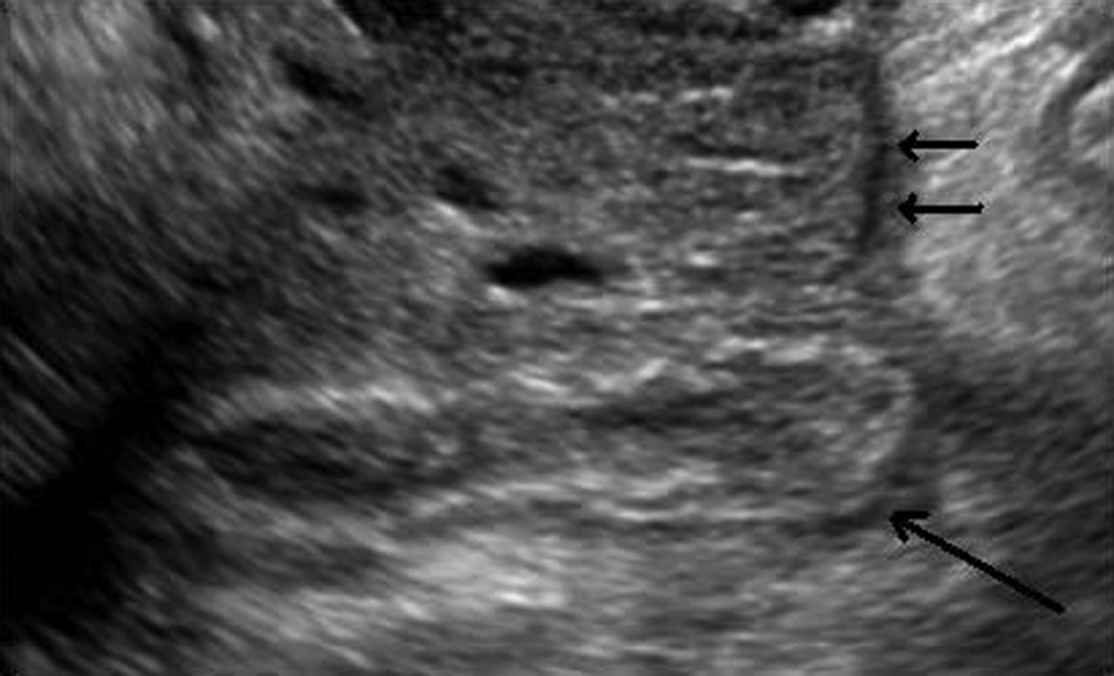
Transvaginal sonogram in a 16-year-old patient shows a thick-walled appendix with lumen distended with fluid (long arrow). A normal right ovary is seen just anterior (short arrows).
